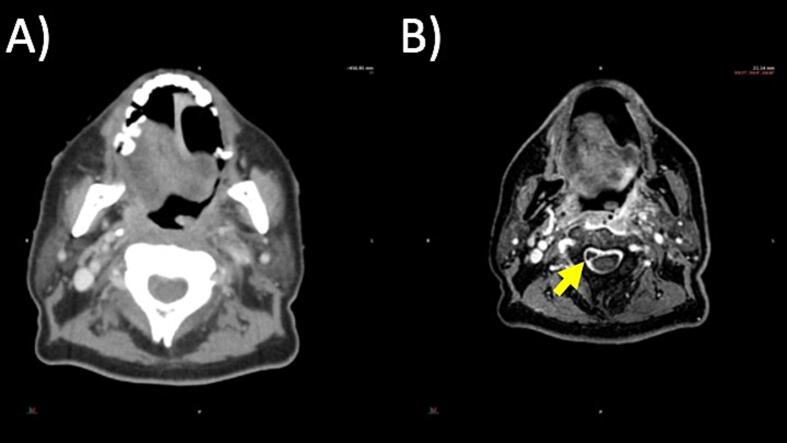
A patient with oropharyngeal squamous cell carcinoma recently treated with transoral resection was noted on MRI sim to have a phlegmon and epidural abscess at the C2-C3 level from iatrogenic diskitis-osteomyelitis. (A) CT and (B) MRI sim images are shown.
